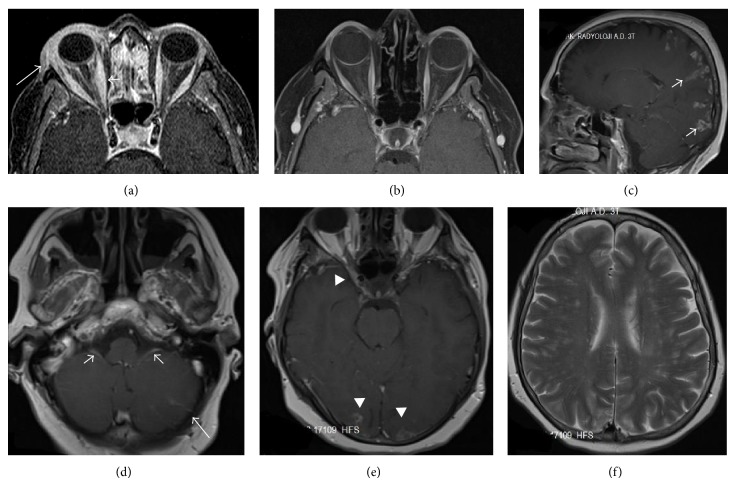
Thickening of the medial rectus muscle (short arrow; right diameter 9.5 mm; left diameter 5.8 mm) with gadolinium enhancement of the right lacrimal gland (long arrow) and periorbital fatty tissue in the axial fat-suppressed postcontrast T1-weighted sequences (a). The lacrimal gland and the medial rectus muscle appear normal in the axial fat-suppressed postcontrast T1-weighted sequences (b). Pial-gyral nodular gadolinium-enhanced lesions (short arrow) are detected in the bilateral occipital and parietal areas in the sagittal postcontrast T1-weighted sequences (c). Pial gadolinium enhancement (long arrow) and diffuse enhancement of the 9th and 10th cranial nerves (short arrow) are observed in both cerebellar hemispheres in the axial postcontrast T1-weighted sequences (d). Pial-gyral nodular gadolinium enhancement was also observed to be adjacent to the right optic foramen and the bilateral occipital area in axial postcontrast T1-weighted sequences (arrowhead) (e). In axial T2-weighted sequences, hyperintense multiple nodular lesions were detected in the bilateral periventricular white matter (f).
